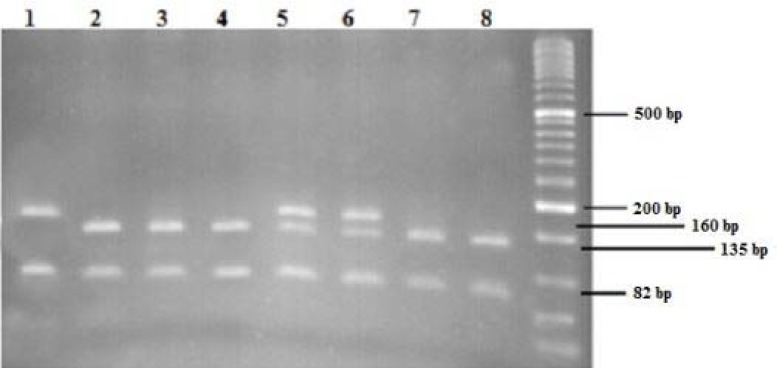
rs12979860 PCR-RFLP genotyping. Digestion of this product with Bstu І in CC individuals produced 3 fragments of 135bp, 82bp, 25bp; 4 fragments of 160bp, 135bp, 82bp , 25bp in CT individuals; and 2 fragment of 160bp , 82bp in TT individuals. Lanes no. 1 was genotyped TT, 2, 3, 4, 7 & 8 were genotyped CC, lane no. 5 & 6 were genotyped CT
